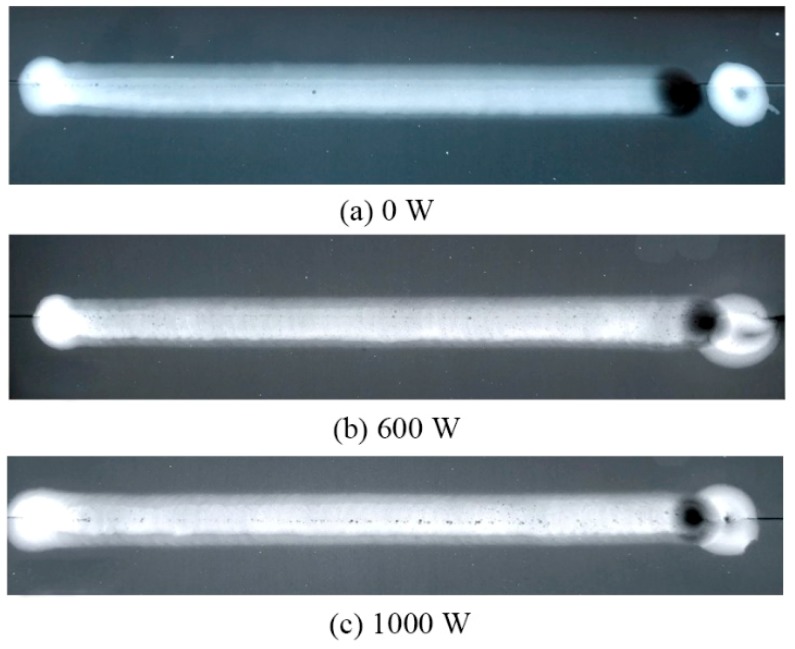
X-ray detection of the joint. Ultrasonic power: (a) 0 W; (b) 600 W; (c) 1000 W.
